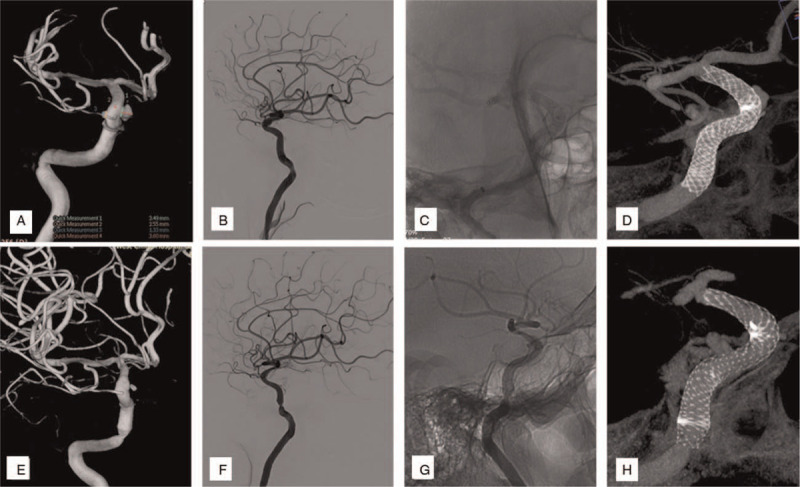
(Case 2): Are images associating shape change of PLED as the cause of ISS. A: shows 2 aneurysms in the right ophthalmic segment of internal carotid artery (ICA). B: shows the lateral view of the parent artery. C: Shows un-subtracted image of implanted PLED intraoperatively. D: is a diluted contrast Xper-CT image of the stent intraoperatively. E: shows a little residual in 1 aneurysm while another was completely occluded on 6-month follow-up images. F&G: Are lateral view of parent artery showing vessel lumen stenosis. H: shows the proximal shape change of the stent on a diluted contrast Xper-CT image.
